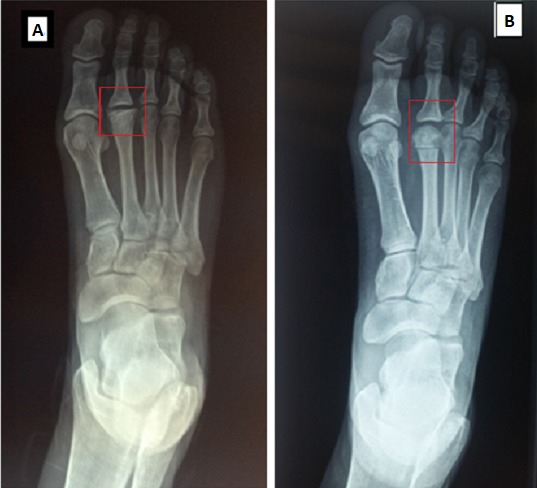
Radiographie standard; (A) Freiberg de la 2ème tête métatarsienne; (B) congruence articulaire après ostéotomie de Gauthier
